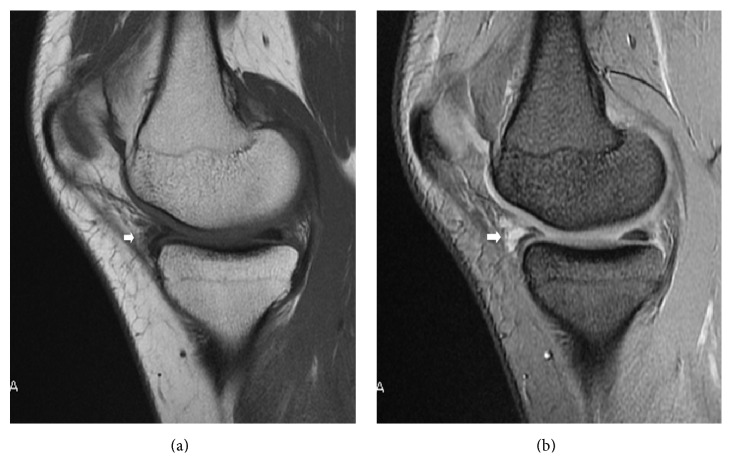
Preoperative magnetic resonance images of the right knee showed a cystic lesion adjacent to the anterior horn of the medial meniscus. (a) Sagittal T1-weighted image and (b) Sagittal T2-weighted image. White arrows indicate the lesion.
